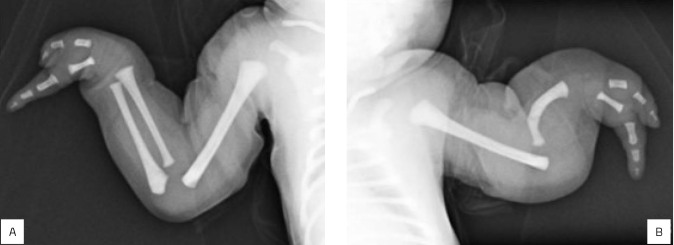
(A) X-ray of the right upper limb corresponding to Bayne’s classification 1 and Cole and Manske’s classification C. (B) X-ray of the left upper limb corresponding to Bayne’s classification 4 and Cole and Manske’s classification C.
